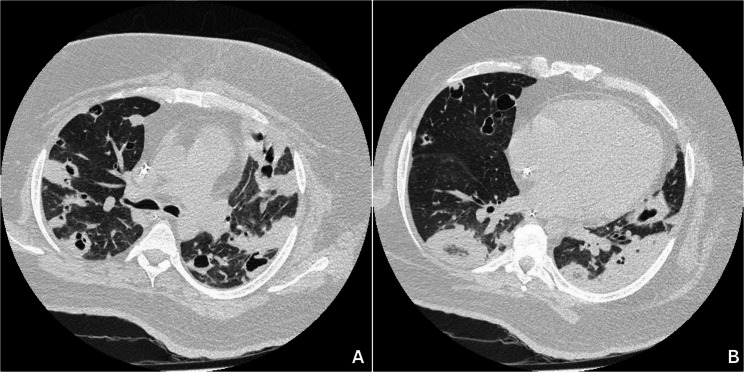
Chest computed tomography. Multiple centrally distributed peribronchovascular consolidations, many with central cavitation, irregular internal septations and dense material. Multiple bilateral pulmonary nodules (<8 mm) with random distribution. No pleural effusion is observed. Right paratracheal lymph nodes appear reactive
